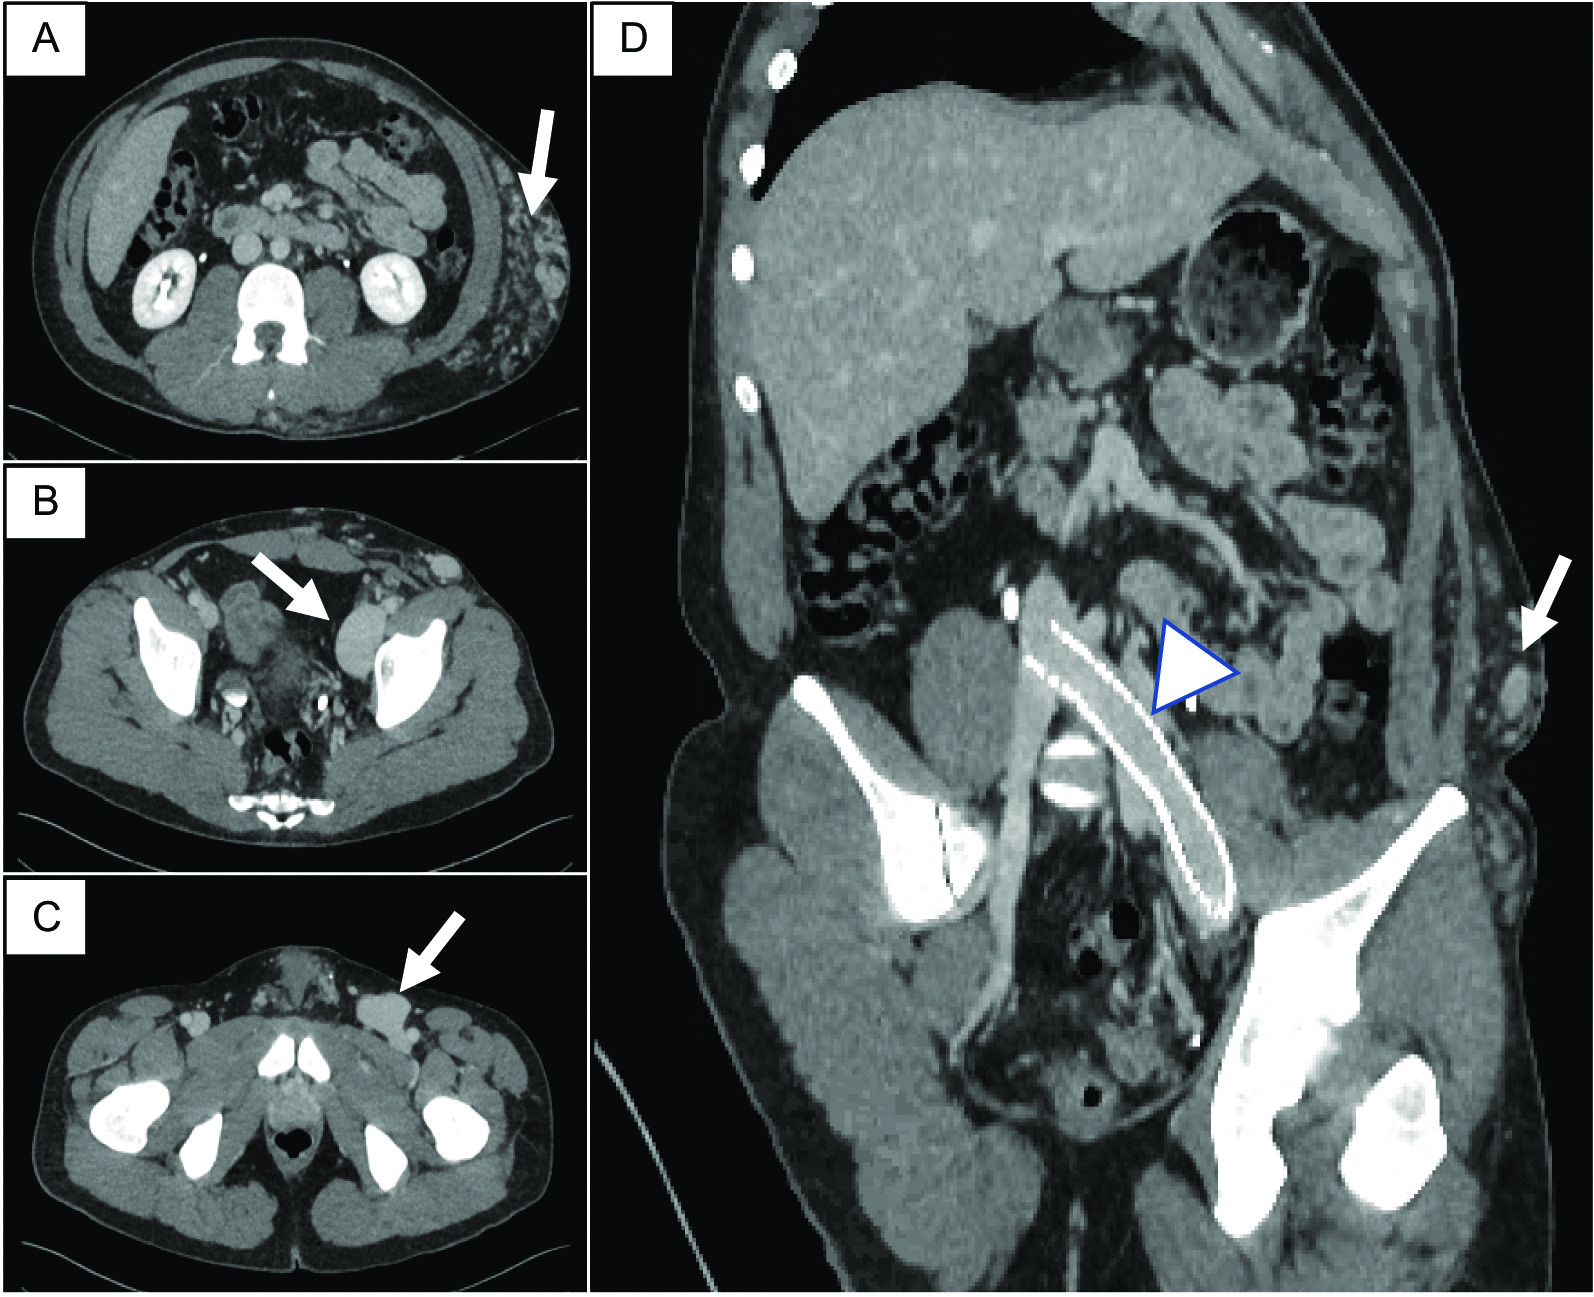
A–C Computed tomography with contrast agent showing the vascular malformation (arrow) and placement of venous stent (D, arrow head)
